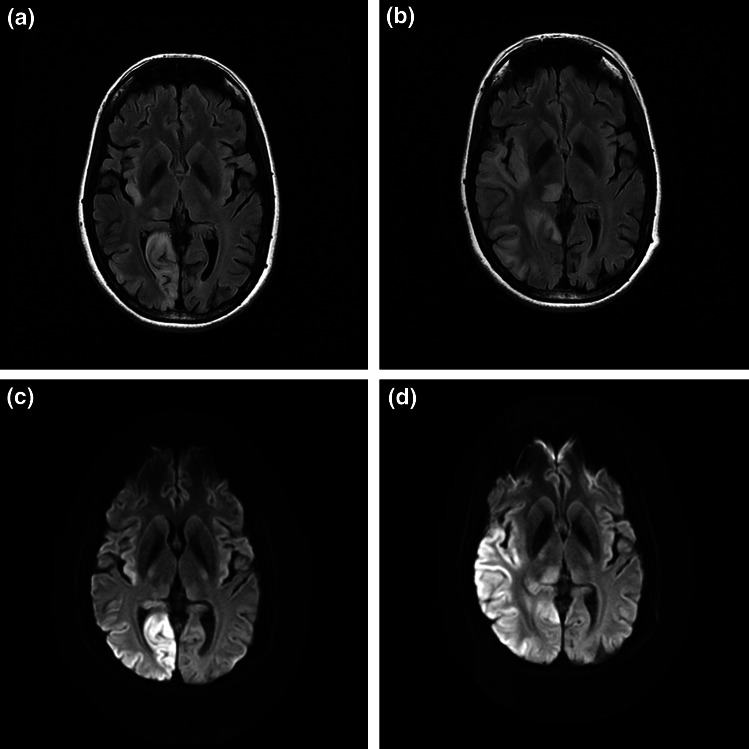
Axial FLAIR (a, b) and DWI (c, d) sequences of MRI head. a and c were performed on admission whilst b and d were performed 8 days later. The stroke-like lesion ‘spread’ from the right occipital lobe to the right temporal lobe and thalamus
